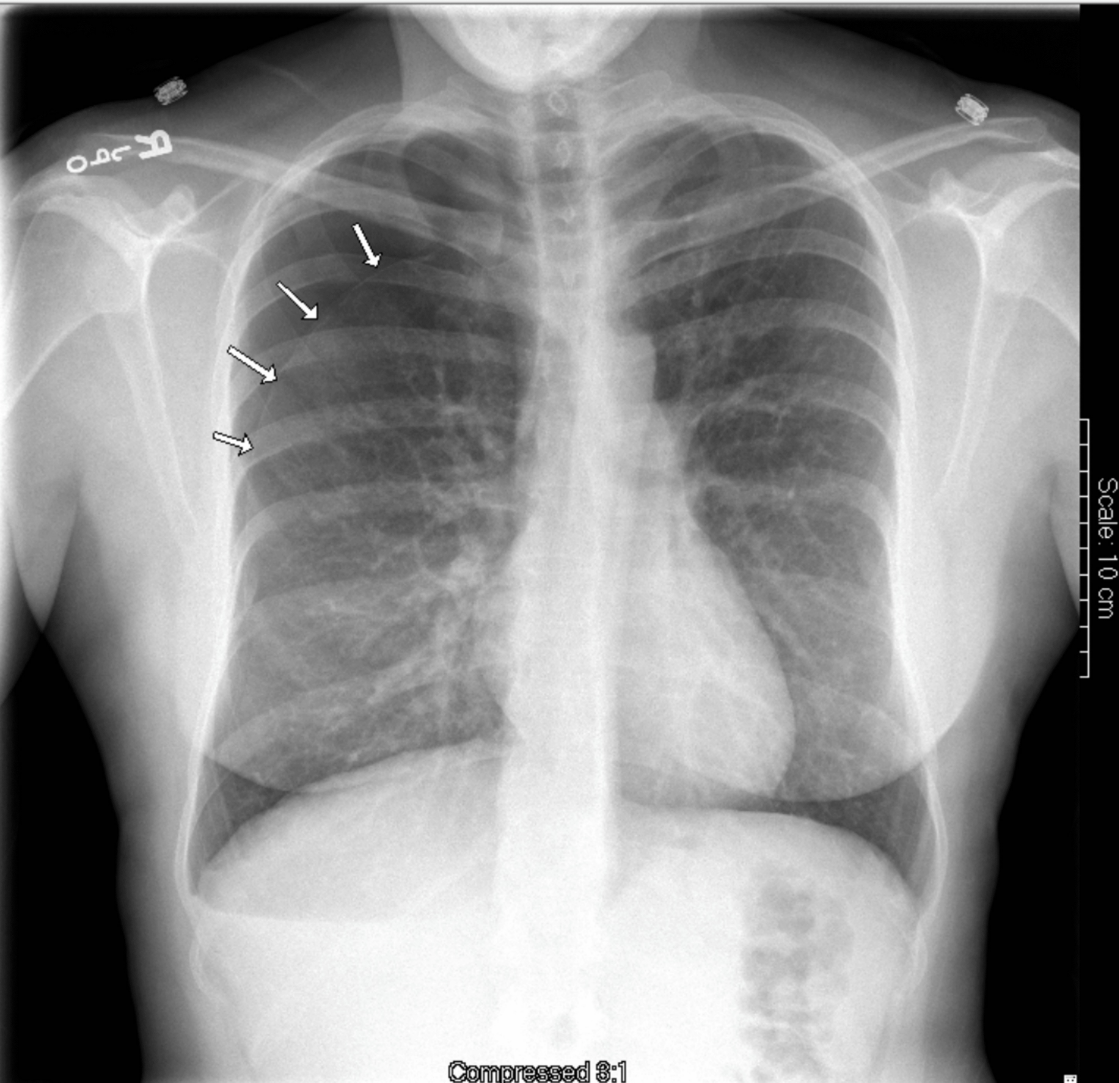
PA chest X-ray on initial presentation There is an appreciable moderate-sized pneumothorax in the right upper lung, as shown by the single arrows PA: posteroanterior
